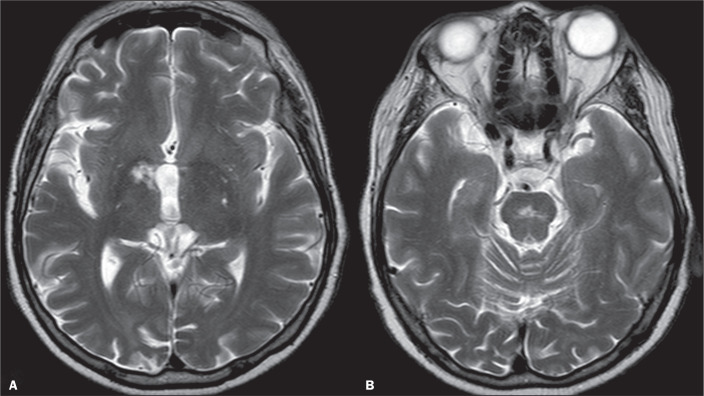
Axial T2-weighted image (A) showing a lesion with a hyperintense signal in the right posterior limb of the internal capsule and in the right ventral thalamus. Axial T2-weighted image (B) showing a hyperintense signal in the midbrain, at the decussation of the superior cerebellar peduncles. On other images (not shown), the lesions do not show enhancement on a contrast-enhanced T1-weighted image and showed markedly hypointense foci on susceptibility-weighted imaging. The patient was in treatment for Behçet’s disease, presenting with ataxia, and the lesions correspond to vascular inflammation related to neuro-Behçet’s disease.
